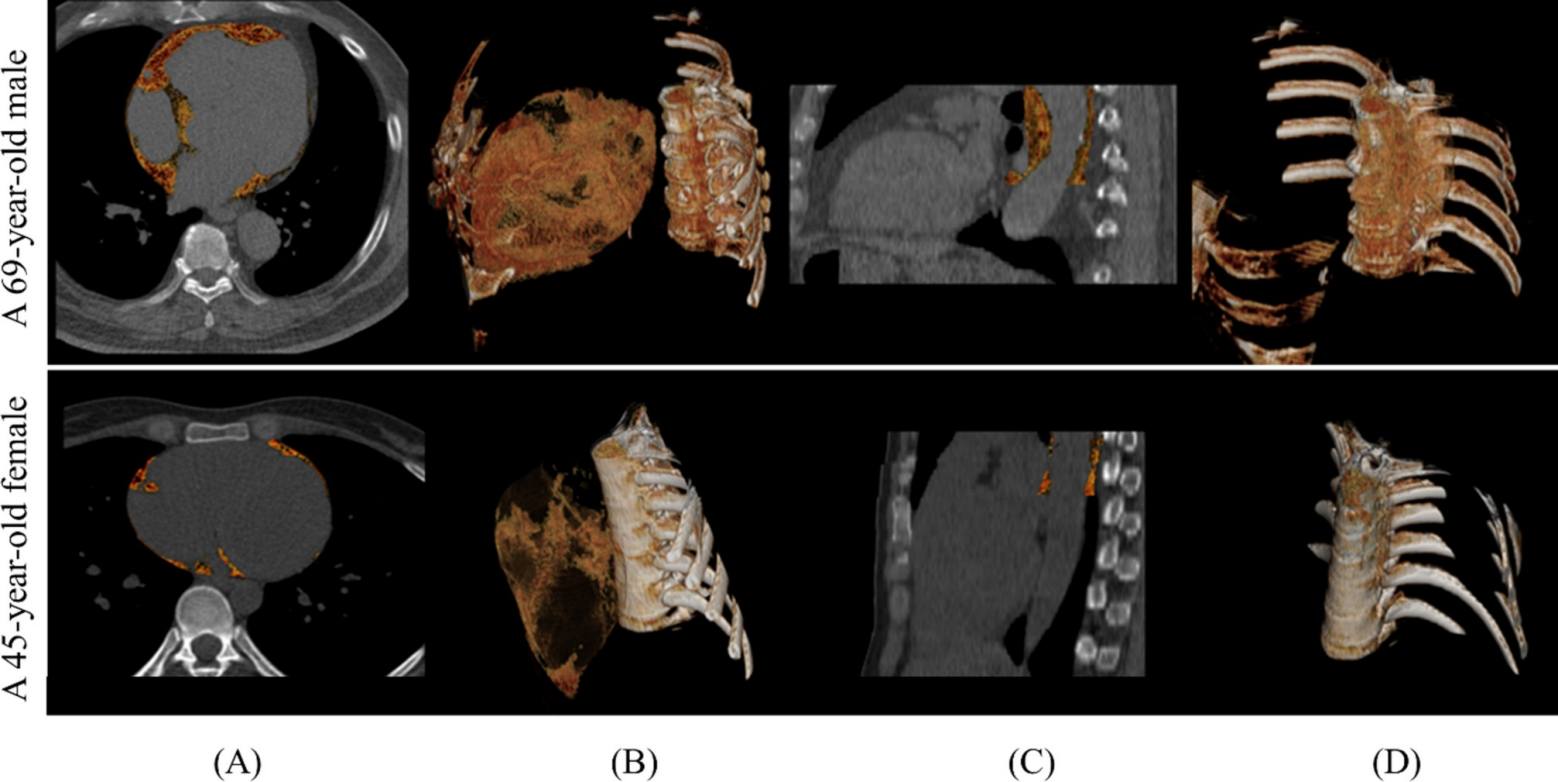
Examples of PCF (A, B) and TAT (C, D) in 2D and 3D computed tomography views. A 69-year-old male with large amounts of PCF (235.8ml), TAT (24.9ml) and the lab data (WBC: 10700/mm^3^, Neutrophil: 9502/mm^3^, NLR: 15.05, monocyte: 481/mm^3^) (First row). A 45-year-old female with small amounts of PCF (18.7ml), TAT (1.24ml) and the lab data (WBC: 3900/mm^3^, Neutrophil: 2211/mm^3^, NLR: 1.702, monocyte: 238/mm^3^) (Second row). PCF: peri-cardial adipose tissue; TAT: thoracic peri-aortic adipose tissue; WBC: white blood cell count; NLR: neutrophil-to-lymphocyte ratio.
